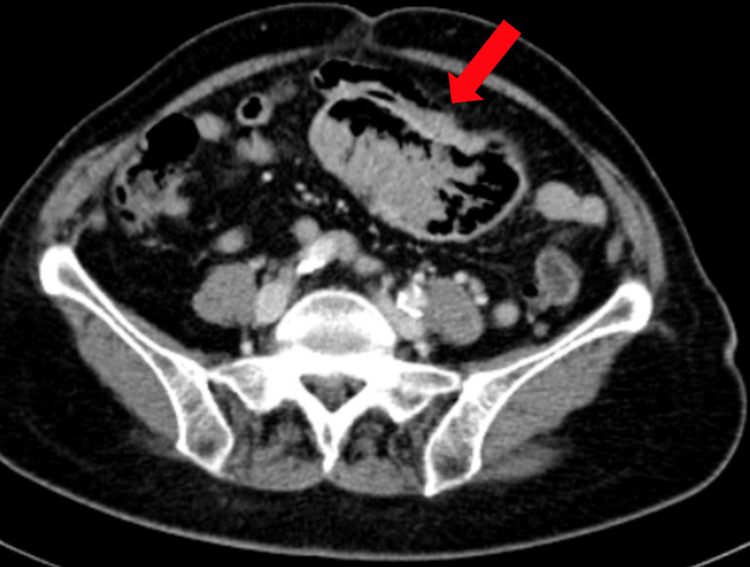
CT scan with oral-rectal water and IV contrast of the abdomen showing a small bowel mass lesion (red arrow) at the mid-abdomen.
